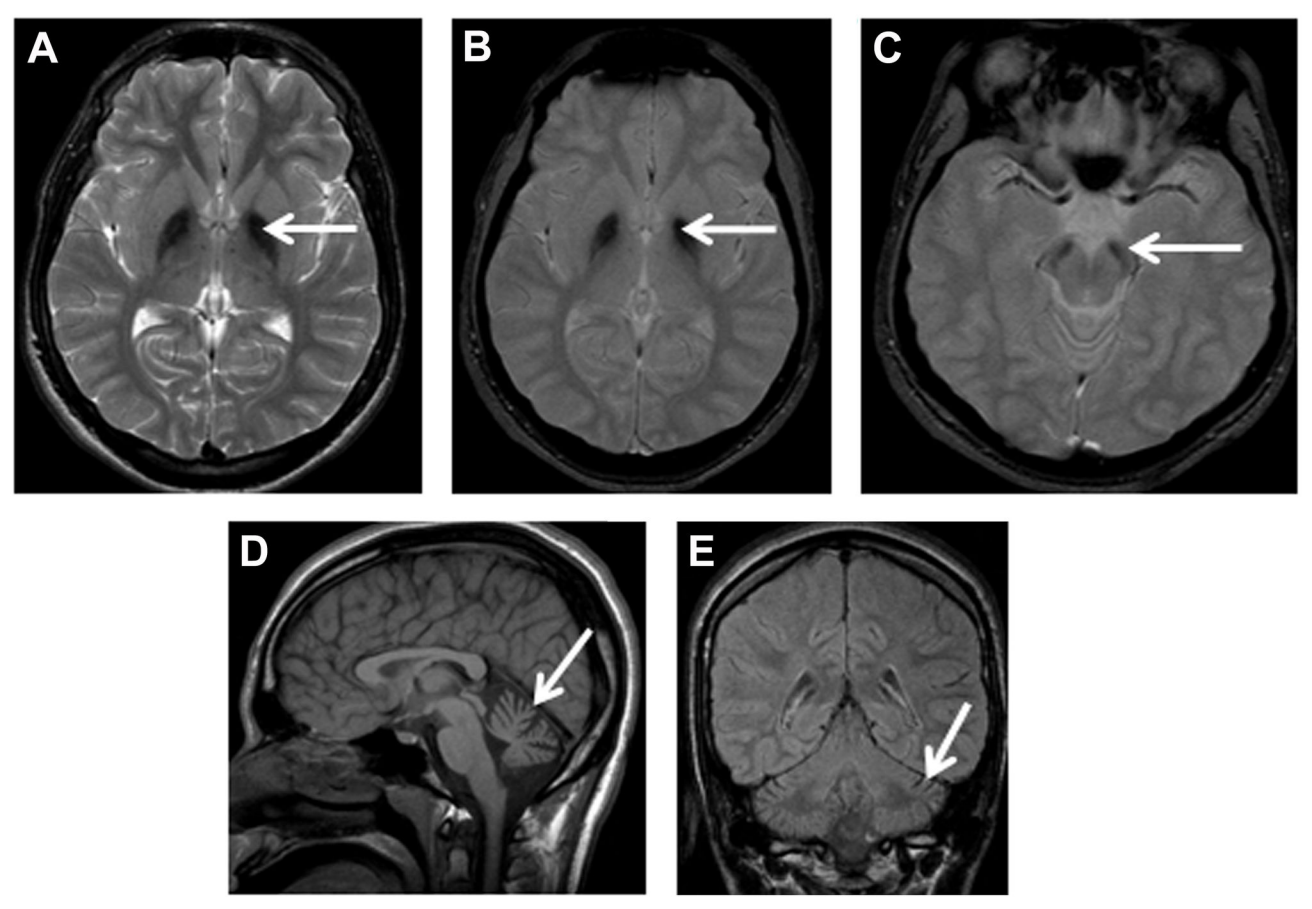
Patient F6 (P3) MRI, age 17 years 5 months. Axial T2-weighted (A), axial gradient (B and C), sagittal T1-weighted (D) and coronal fluid-attenuated inversion recovery (FLAIR, E) sequences highlight iron deposition as reduction in signal intensity in the globus pallidi (arrows in A and B) and *substantia*
